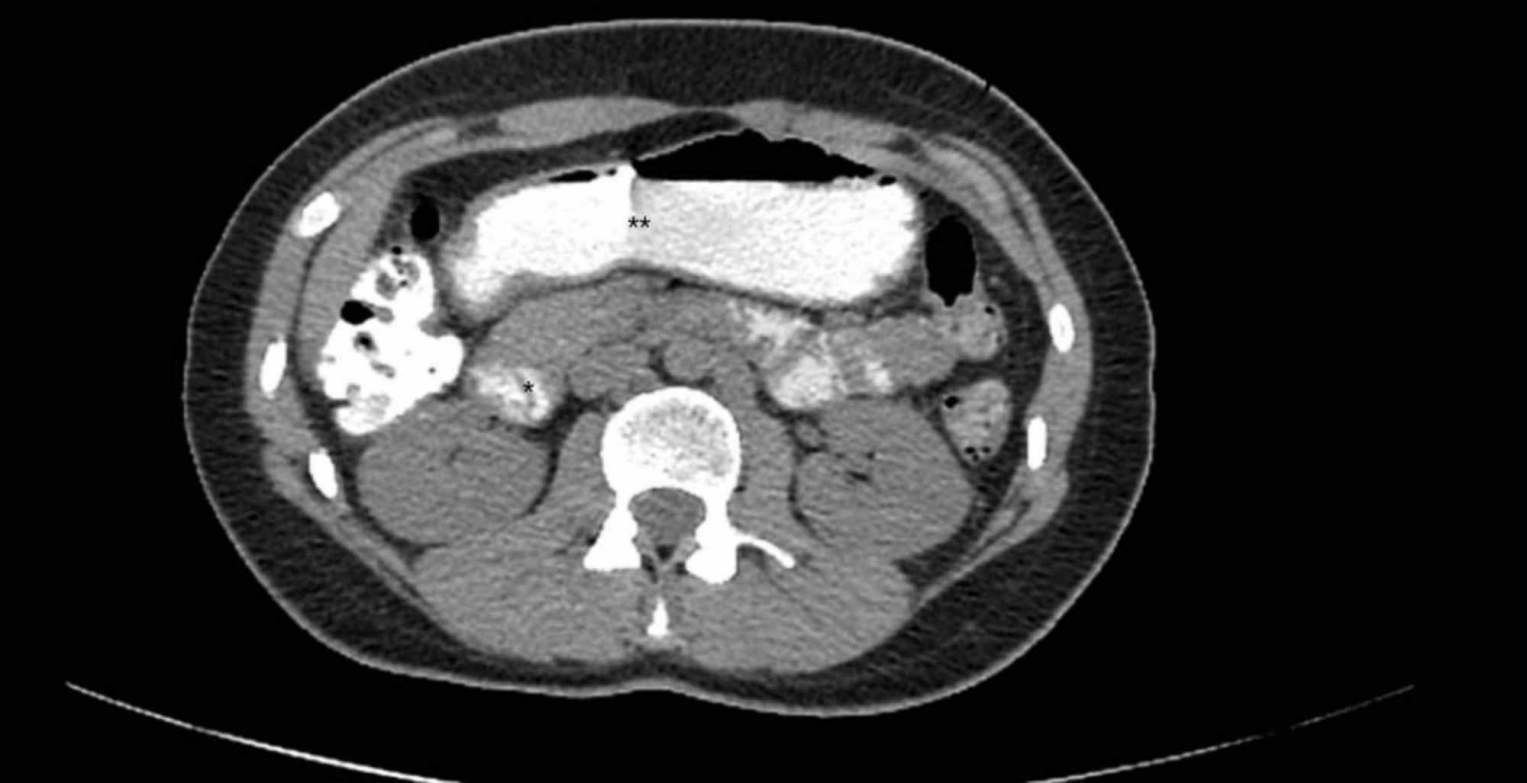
Computed tomography (CT) of the abdomen with oral contrast showing gastric distension () and duodenal compression with retention of contrast (*)
